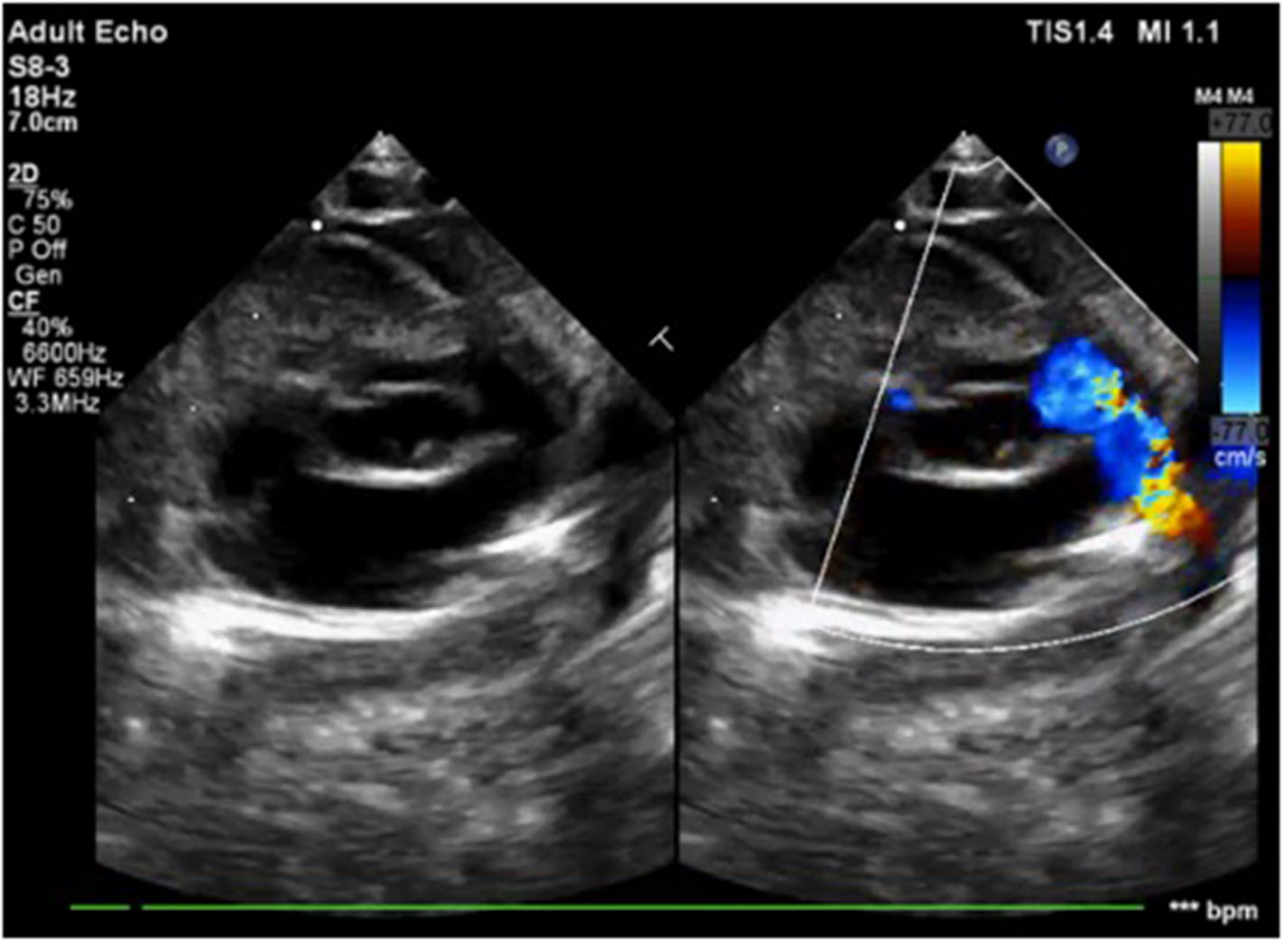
Post-procedure echocardiogram showing good antegrade flow across the pulmonary valve
